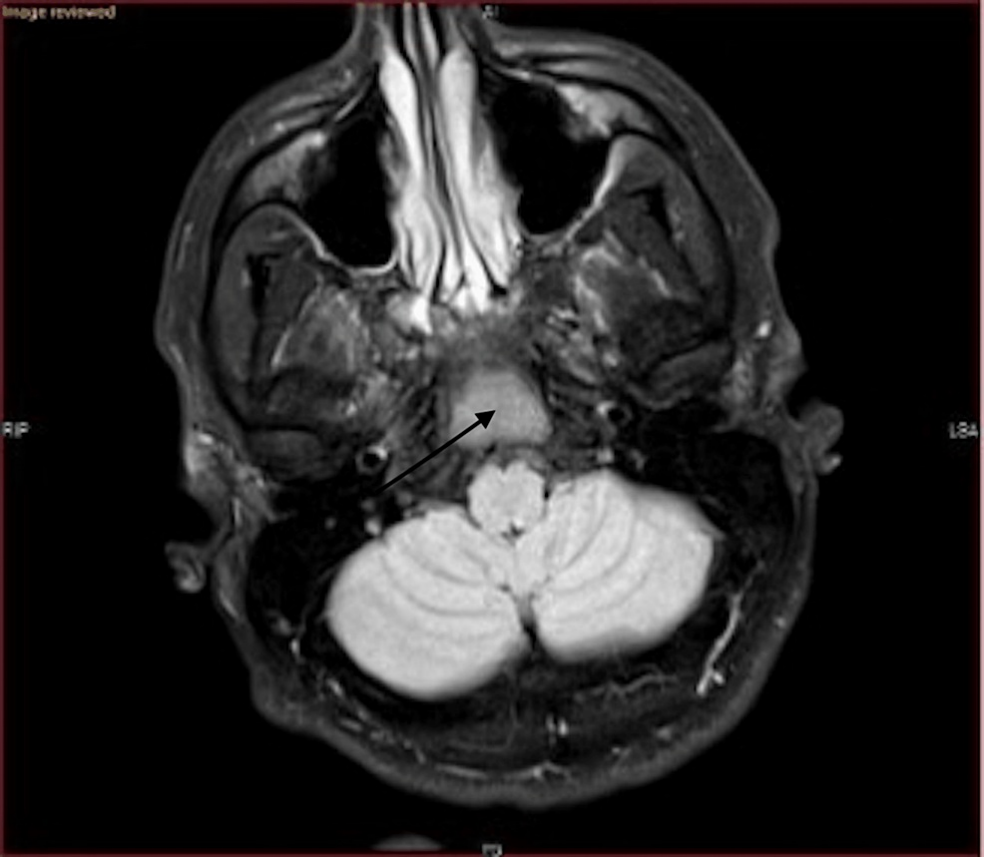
Mass in the clivus of low, hypointense signal intensity on T1-weighted image
